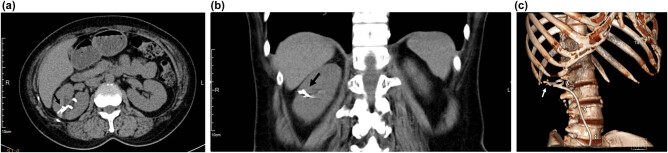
Computed tomography image of the intrarenal foreign body (arrow) and its relationship with right double J ureter stent (arrowheads). (a) Transverse cut, (b) coronal cut, and (c) reconstruction.
